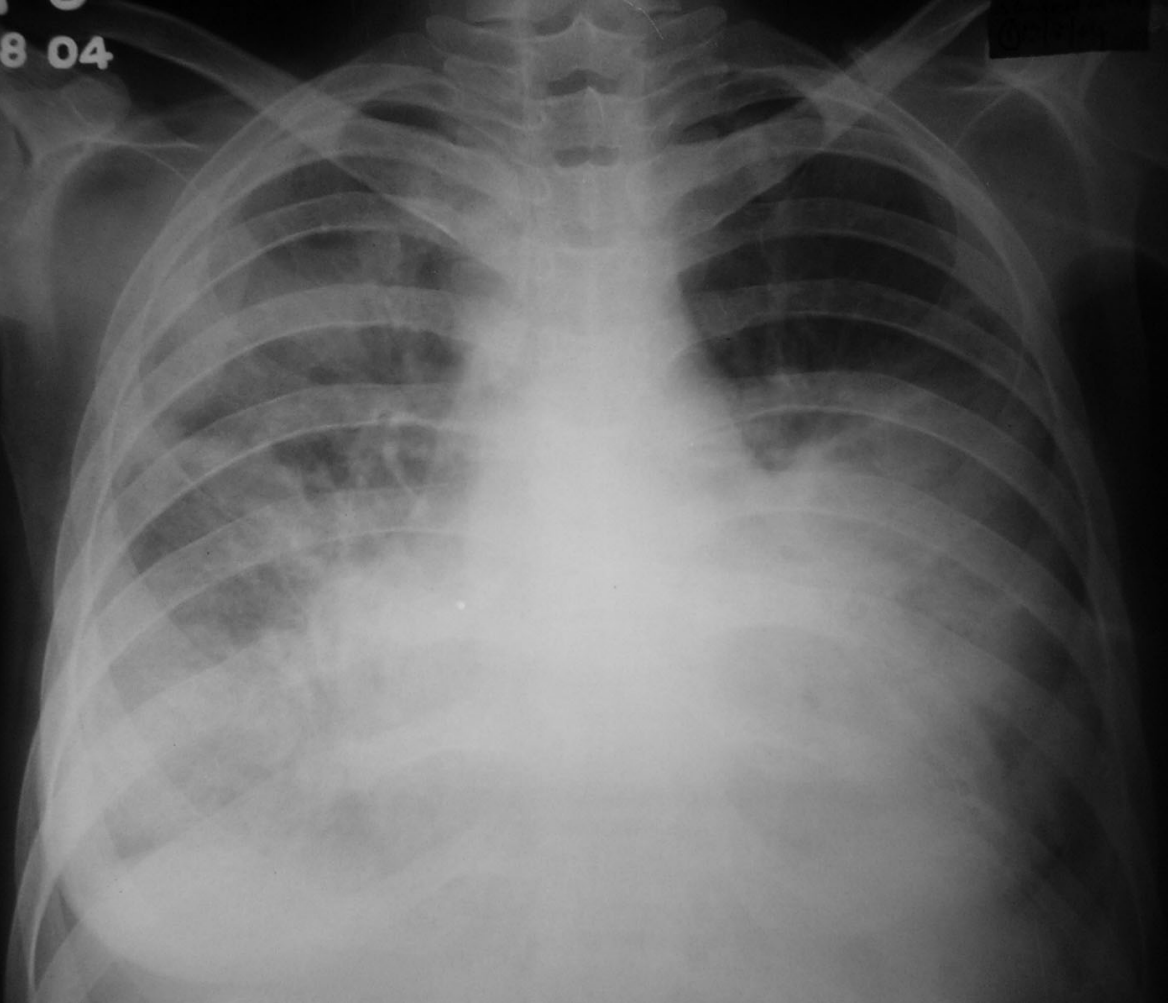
Chest radiograph bilateral alveolar opacities and cardiomegaly.
